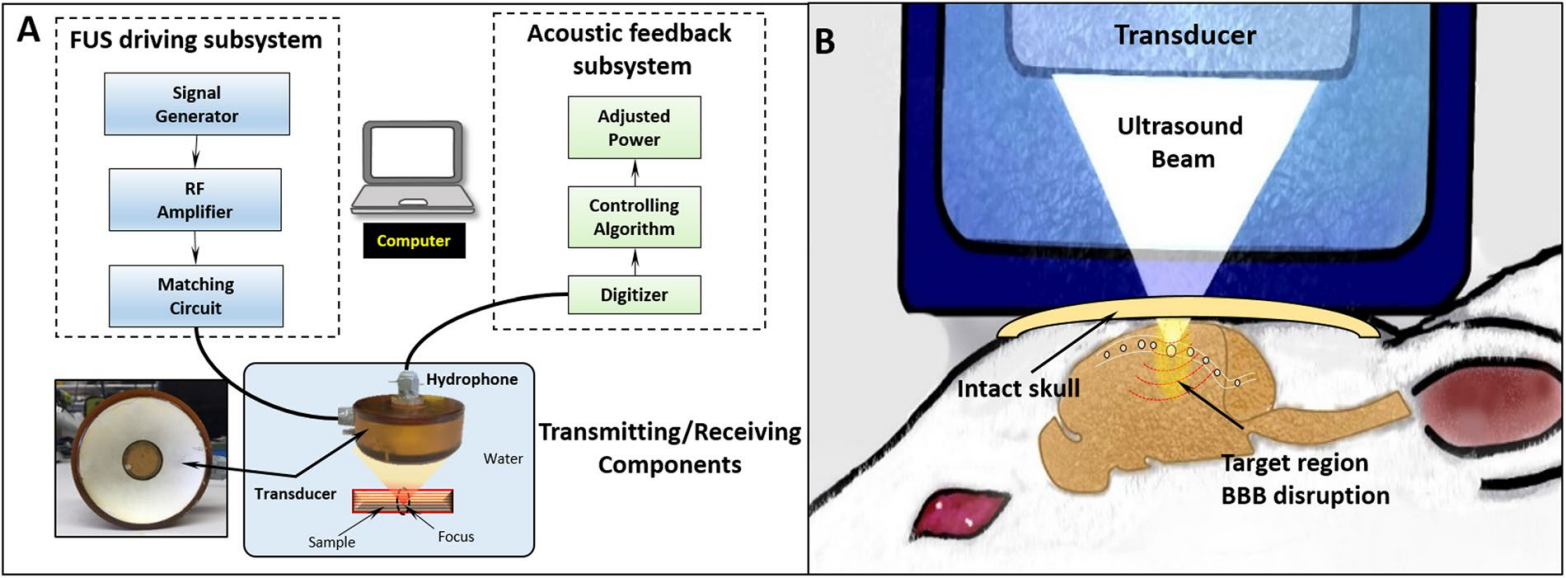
Acoustic feedback control ultrasound system. (A) The system components include an ultrasound transmitting subsystem and an acoustic feedback control subsystem. The ultrasound transmitting system is comprised of a signal generator, RF amplifier, impedance matching circuit, and a single element transducer; The feedback control system includes a hydrophone with a central frequency of 0.75 MHz, digitizer, and an algorithm which can adjust the ultrasound pressure based on the detected acoustic emissions from the stimulated bubbles. (B) Transcranial *in vivo* setup. Details of the system could be found in our previous publication^22^.
